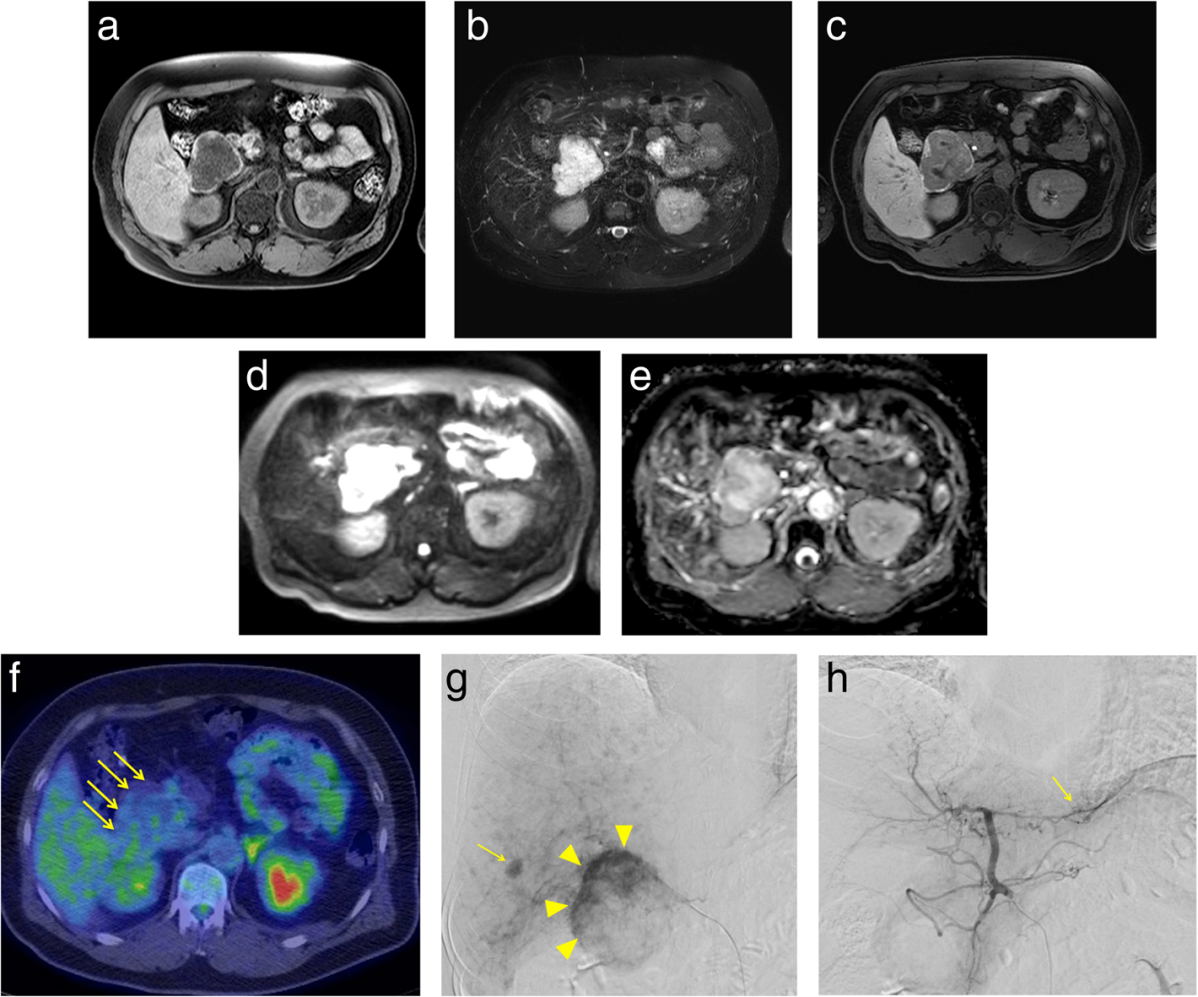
Magnetic response imaging (MRI), [^18^F]-fluorodeoxyglucose positron emission tomography (FDG-PET) and hepatic arteriography. MR images showing low-signal intensity on T1-weighted images (a) and high-signal intensity on T2-weighted images (b). EOB-MRI revealed a hypointense mass in the hepatobiliary phase (c). The ADC mean of DW-MRI in the mass was 2.33 × 10^−3^ mm^2^/s (d, e). There is no accumulation of [^18^F]-FDG (f). Hepatic arteriography showing hypervascular masses in S1, S6 (g), and S2 (h) of the liver; there is no pooling
